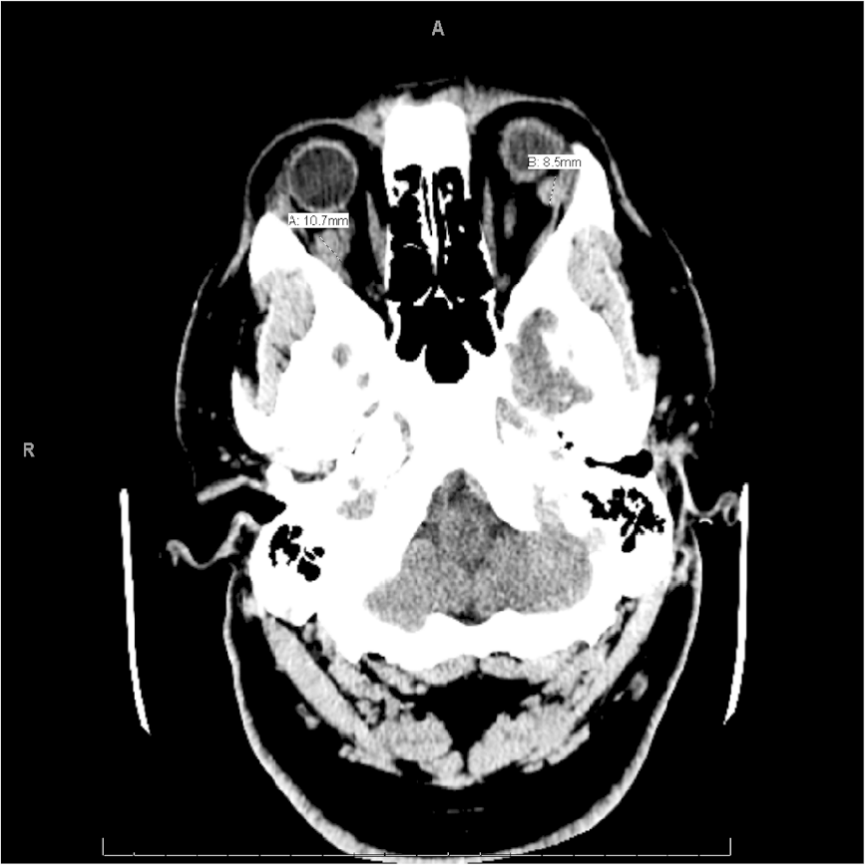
Computed tomography scan of the head showing broad-based multiple bilateral retro-orbital masses along the medial side of the lateral rectus muscle, the largest one measuring 10 mm
